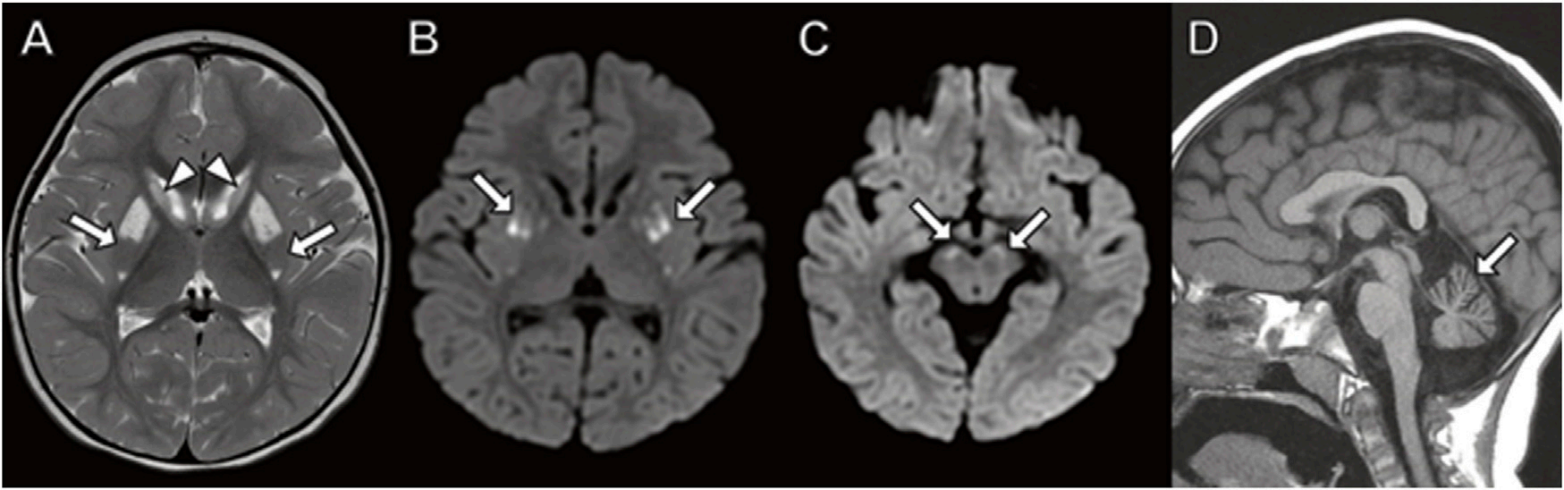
Brain MRI in 22-month-old girl with *SERAC1* mutation. (A) Axial T2 image demonstrates abnormal signal throughout the basal ganglia with sparing of the dorsal putamen (arrow) resulting in a finding described as the “putaminal eye”. There is abnormal signal and atrophy of the caudate heads (arrowheads) and globus pallidus. (B,C) Axial diffusion-weighted images with restricted diffusion in the putamen ((B) arrows) and substantia nigra ((C) arrows). (D) Prominent cerebellar folia indicating cerebellar atrophy (arrow) is present on sagittal T1-weighted image.
